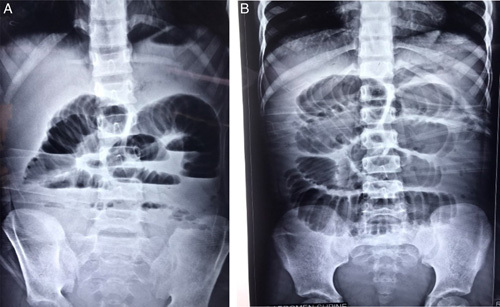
(A) Erect X-ray: abdomen showing air fluid level suggesting small bowel obstruction. (B) Supine X-ray: abdomen showing distended small bowel loops involving whole small bowel.
